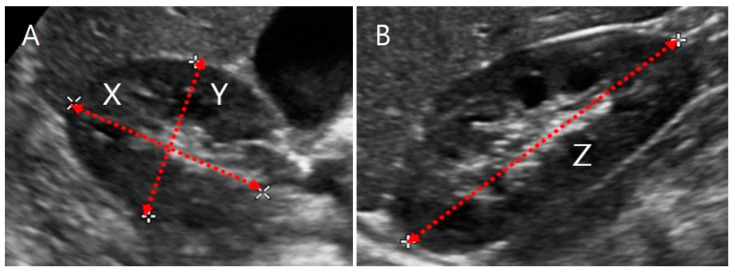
Ultrasound image of a kidney. (A) Transverse section. X is the maximum depth of the kidney parallel to the hilum, and Y is the maximum width perpendicular to X. (B) Coronal section. Z is the maximum bipolar length.
